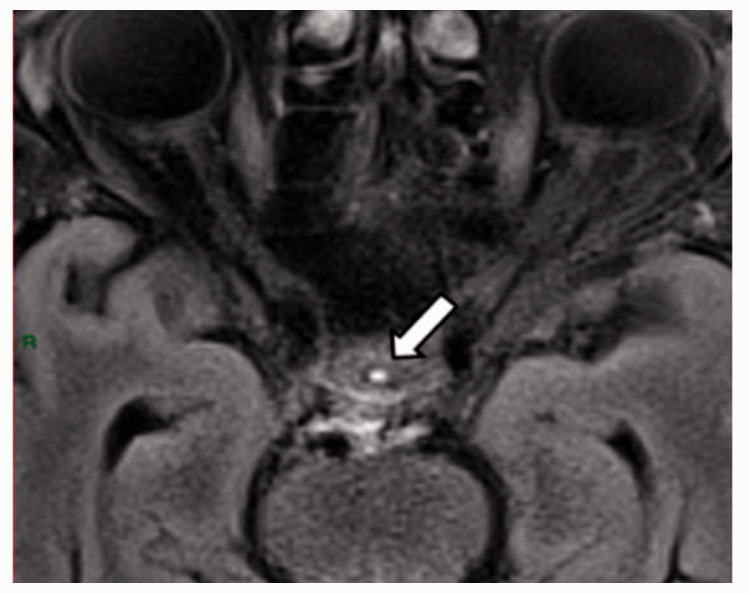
The white bar in the column ‘plane of measurement’ indicates location of scan slice, in the orientation indicated in the column ‘projection’ and shown in the column ‘example images’. In the ‘example images’ the arrows indicate the meningeal lymphatics seen as punctate, linear, or sheet hyperintensities on T2 FLAIR post-contrast that were measured by placing ROIs. For the jugular foramina, superficial cortical perivenous spaces, and normal appearing white matter, ROIs are indicated by circles.
